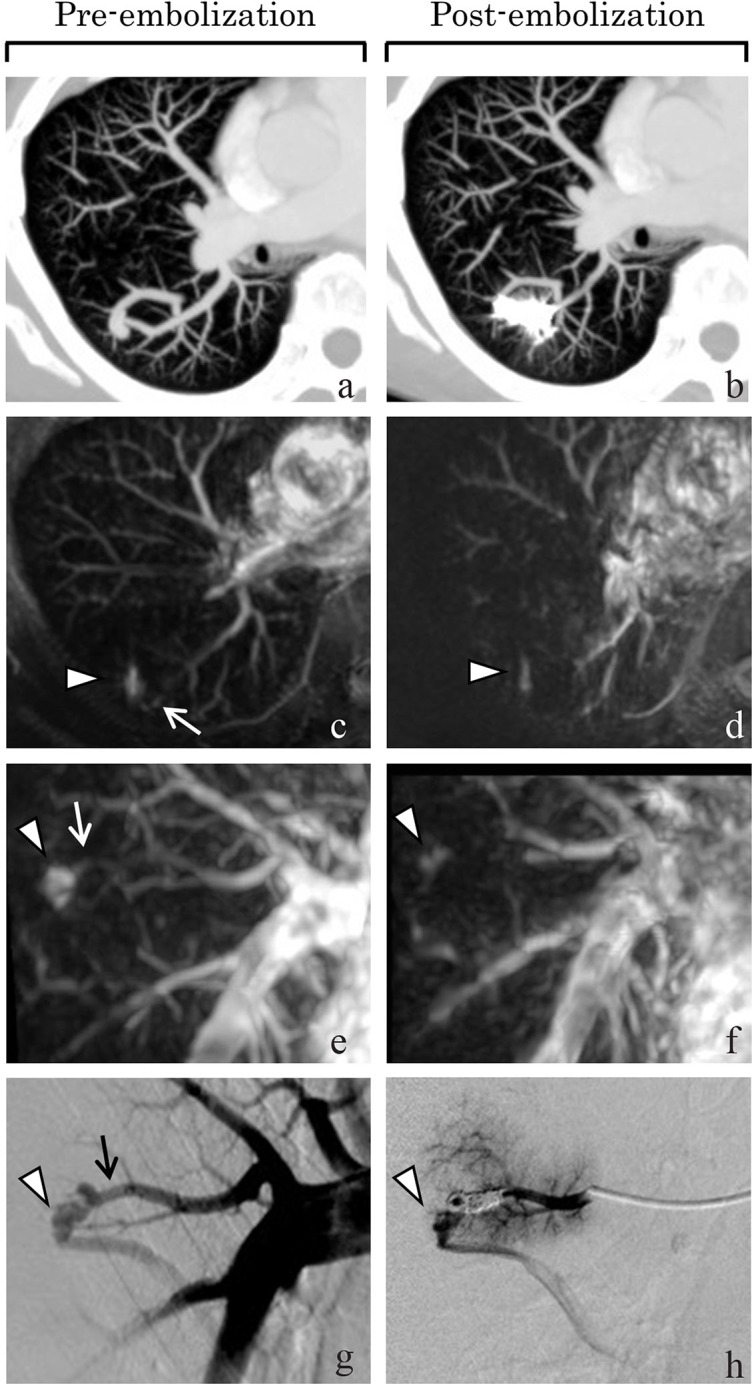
A 53-year-old man with a solitary simple type pulmonary arteriovenous malformation (PAVM) in the right upper lobe. Arrows and arrowheads indicate the feeding artery and aneurysmal sac, respectively. On contrast-enhanced computed tomography (CECT) (thin-slab maximum intensity projection [MIP]) (a), non-contrast-enhanced magnetic resonance angiography with time-spatial labeling inversion pulse (time-SLIP MRA) (c: axial image, e: coronal image), and digital subtraction angiography (DSA) (g) before embolization, a simple type PAVM connecting the segmental artery of upper lobe is seen. On CECT obtained at 6 months after embolization, the size and contrast effect of the aneurysmal sac is unclear because of prominent streak artifacts (b). On time-SLIP MRA, the aneurysmal sac appears at the same site as before embolization (d: axial image, f: coronal image). Recanalization was confirmed by DSA (h).
